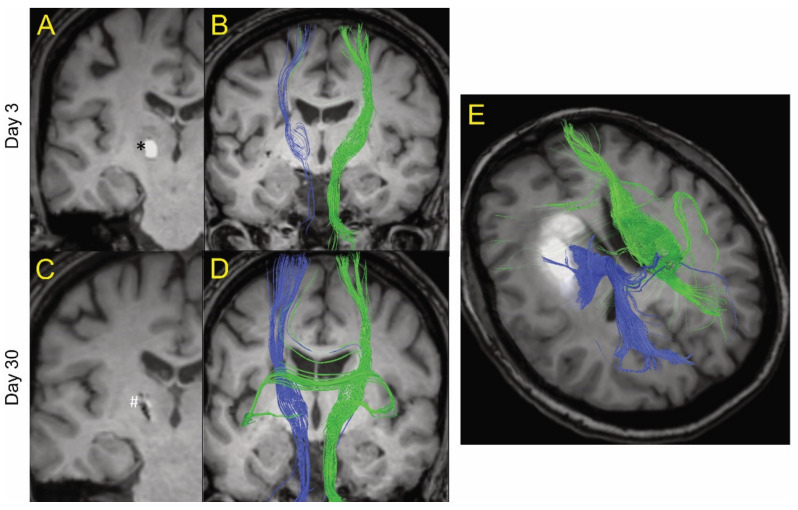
Example of MRI tractography of intracerebral hemorrhage patients. The images are postprocessed 2D axial images with projected WM fibers in 3D in a cranio–caudal direction. One patient (age 50s) is shown at day 3 (A and B) and day 30 (C and D) after ictus. Blue fibers are ipsilateral and green fibers are contralateral to the hematoma. At day 3, there was a general decrease in the fiber tracts (blue) ipsilateral to the hematoma (shown as * in A) compared to contralateral tracts (green). However, there was evidence of some white matter fibers crossing the hematoma. At day 30, the hematoma has largely resolved (shown as # in C) and there was a recovery of the ipsilateral fiber tracts. (E) MRI tractography of a patient (age 60s) with a larger hematoma (19.4 mL) at day 14. It too showed evidence of white matter tracts crossing the hematoma.
